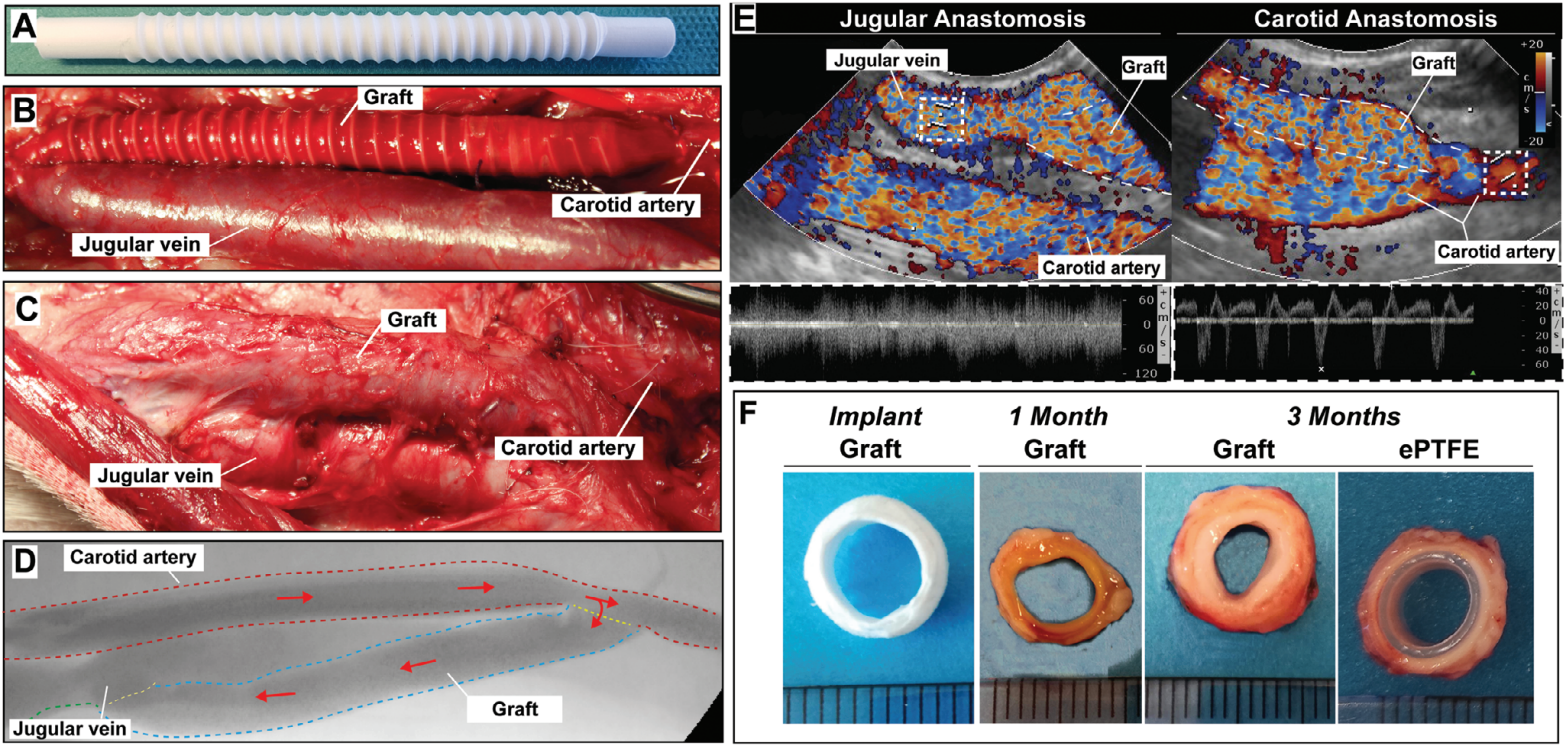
Graft implantation, visualization and explantation. A,B) Synthetic grafts are implanted between the carotid artery and jugular vein end‐to‐side in a straight configuration. C) After 1 and 3 months flow is visualized by D) angioCT and E) color Doppler and the grafts are explanted. F) Cross sections of the grafts at implantation and explantation show the rate of tissue formation and remodeling (scale 1 mm).
